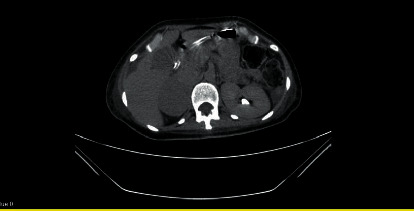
Abdominal CT scan of the patient shows heterogeneous right adrenal mass.
